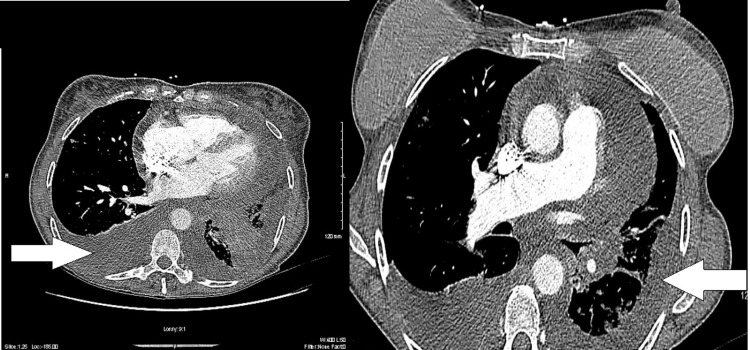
CT chest showing bilateral pleural effusions, loculations, and a moderate-sized pericardial effusion.
